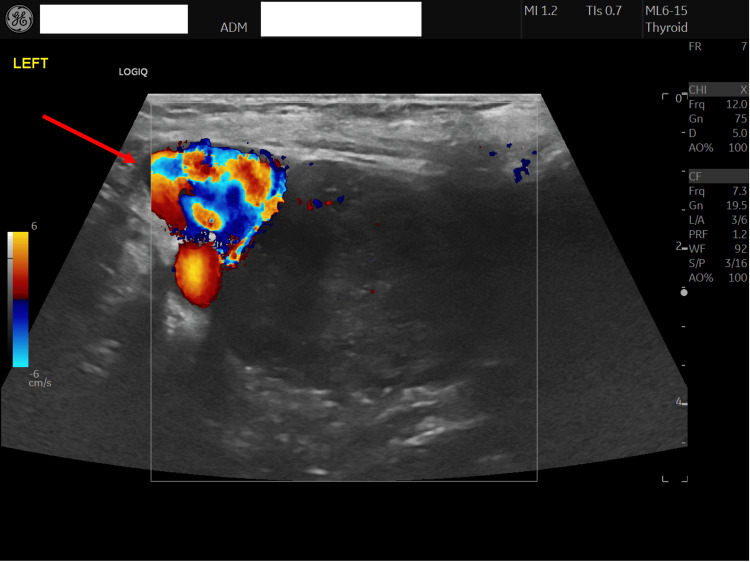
Thyroid ultrasound performed two months after the diagnosis of GD A 37 mm isoechoic nodule with peripheral blood flow is demonstrated in the left thyroid lobe (arrow), classified as U2. GD, Graves’ disease
